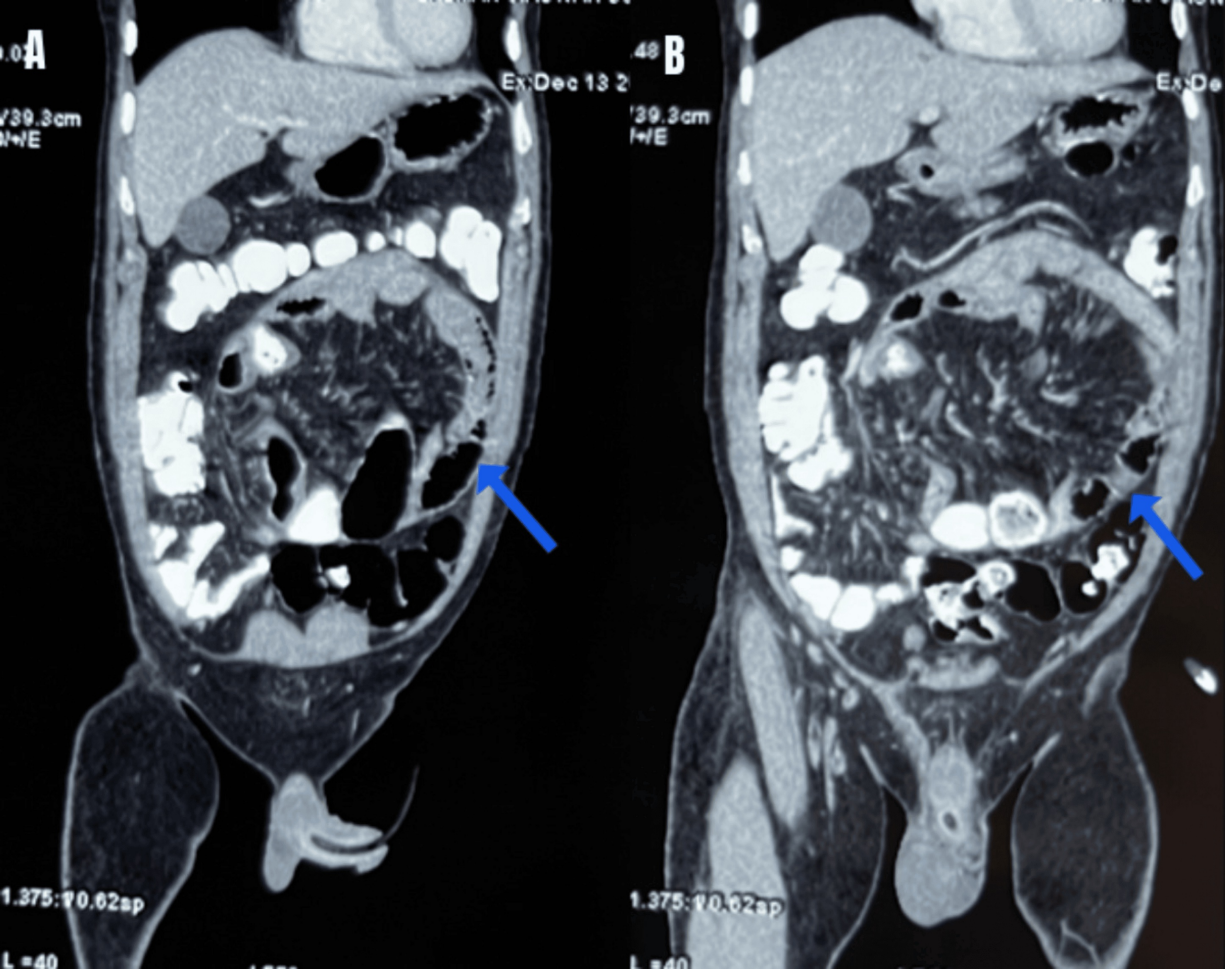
CT abdomen in the coronal plane (A and B) showing membrane (blue arrow) surrounding small bowel loops suggestive of cocoon formation
